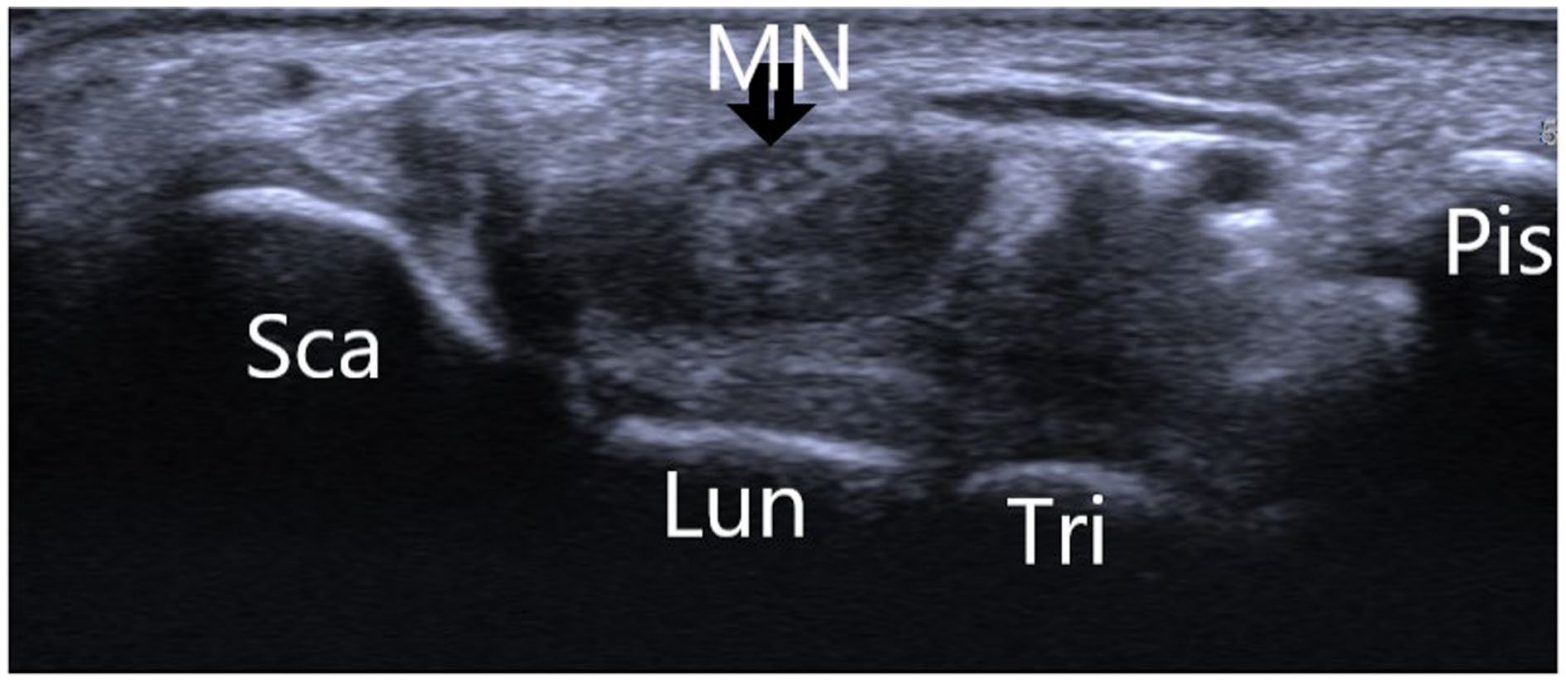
Showing the normal wrist B-ultrasonography image of a 30-year-old asymptomatic male volunteer. *Sca, scaphoid bone; Lun, lunate bone; Tri, triquetral bone; Pis, pisiform bone; MN, median nerve.
